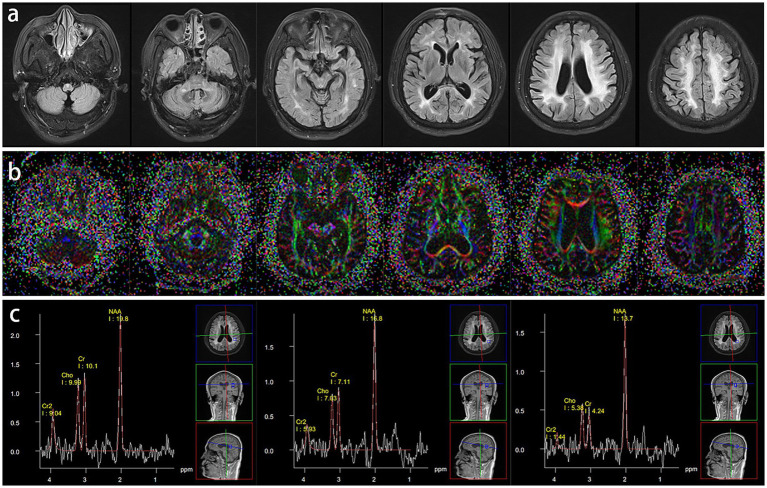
Brain MRI (A), DTI (B), and MRS (C) of the proband.
